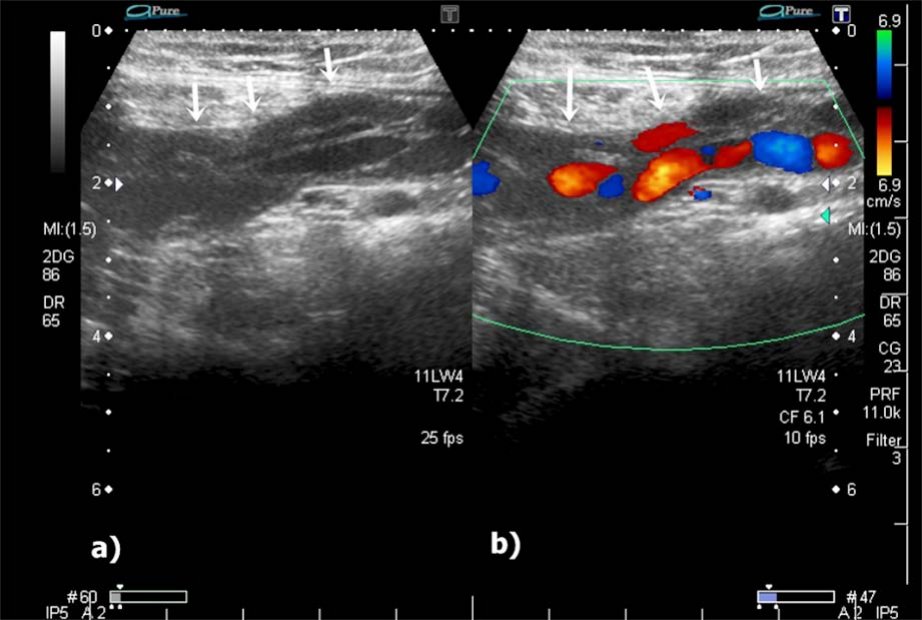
Gray scale and colour Doppler ultrasonography (US) reveals dilated, tortuous channels (white arrows) at the left inguinal region (a, b).
